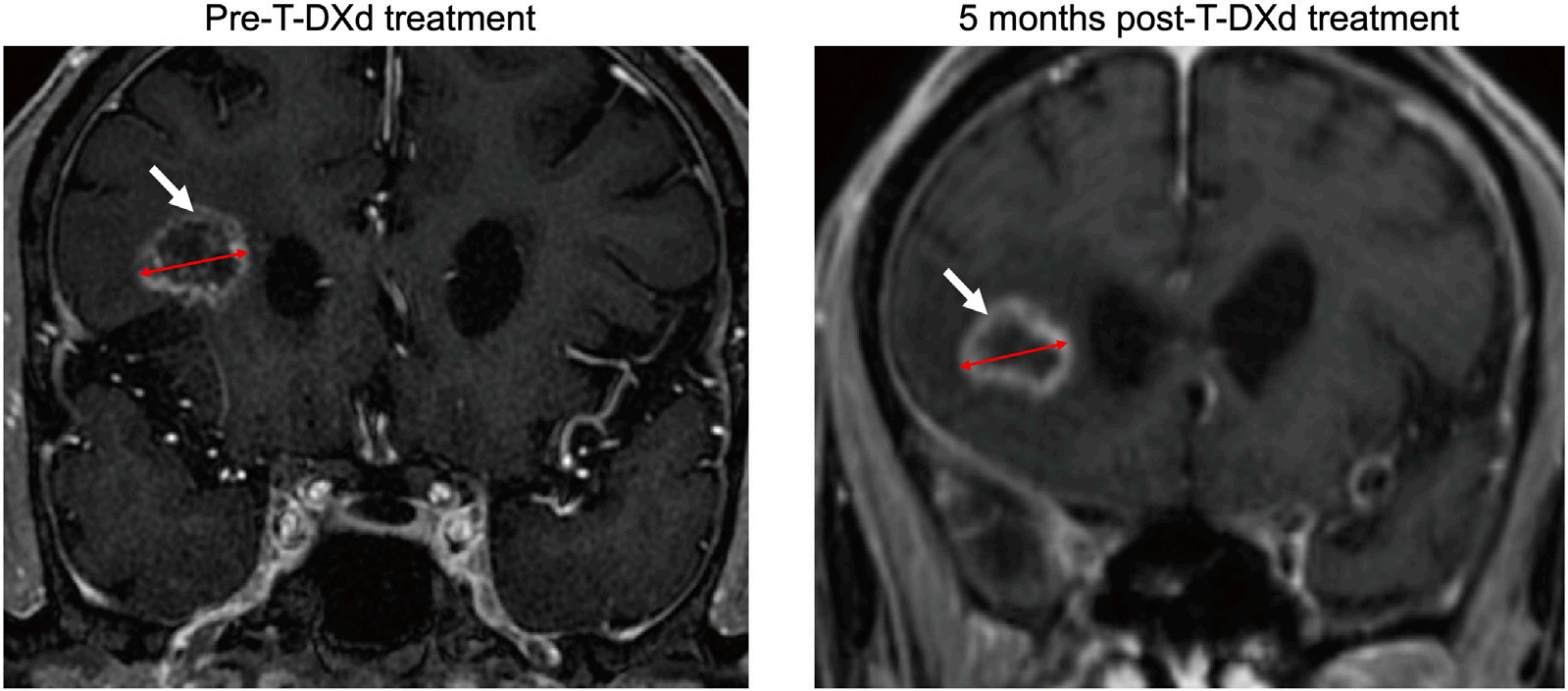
Magnetic resonance imaging of brain metastasis (white arrows) before and after five months of Trastuzumab Deruxtecan treatment. The red double-headed arrows indicate the longest diameter of the lesion.
